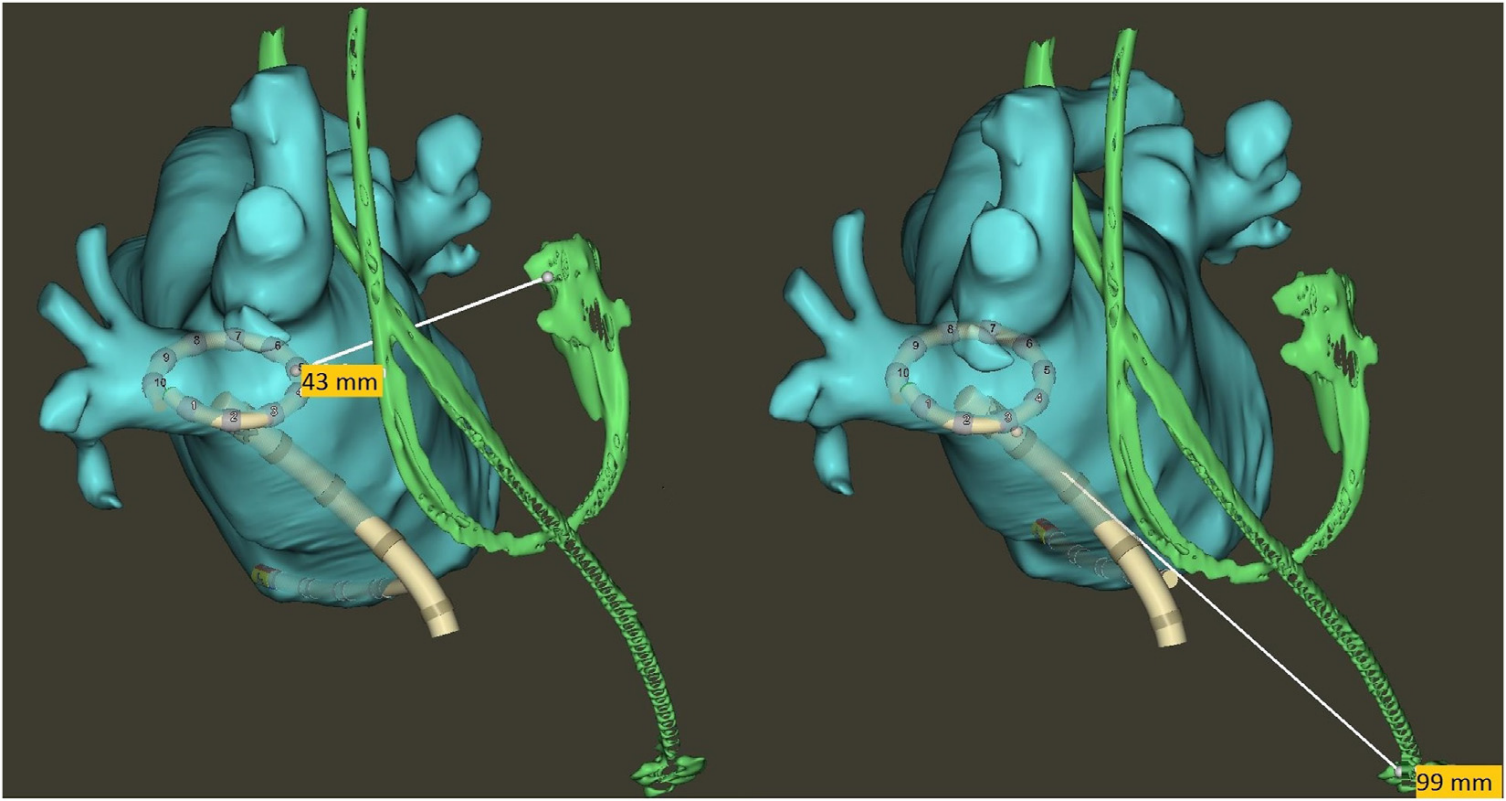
Computed tomography reconstruction of the left atrium and pacemaker leads demonstrating the proximity of the leads to the VARIPULSE pulsed field ablation catheter (Biosense Webster) in the right inferior pulmonary vein (right anterior oblique view). Distance to the right atrium (left) and right ventricle (right) lead tips measured is shown.
